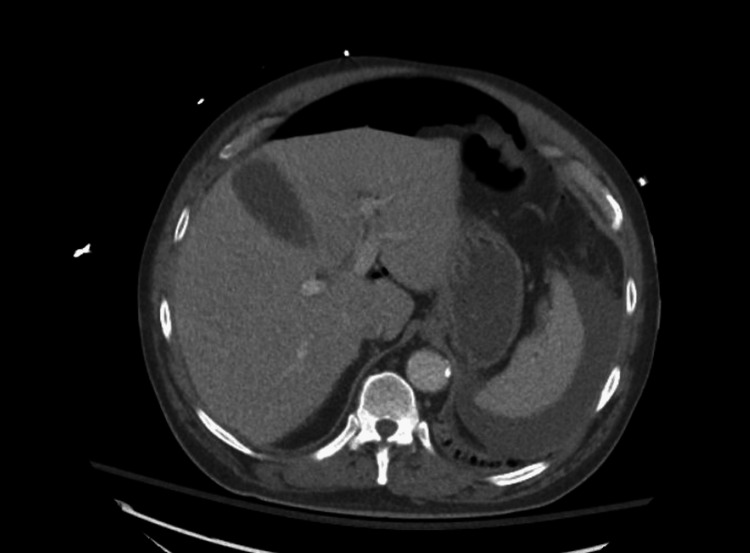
CT abdomen and pelvis with contrast - image 3 Pneumoperitoneum is seen CT: computed tomography
